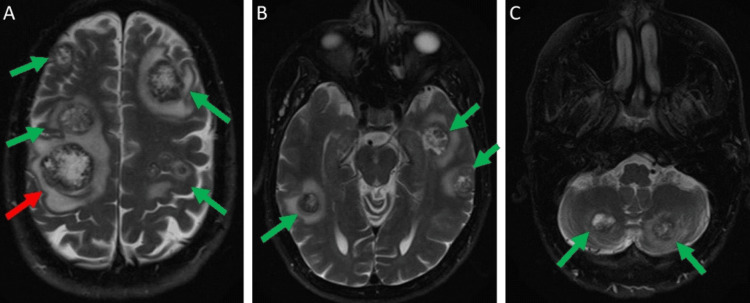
Initial MRI brain with IV contrast T2-weighted images. (A) Multiple brain lesions (green arrows) with significant surrounding edema involving bilateral frontal and parietal lobes, with the largest lesion measuring 2.9 cm in the right frontal lobe (red arrow). (B) Multiple brain lesions with surrounding edema (green arrows) involving bilateral temporal lobes. (C) Brain lesions (green arrows) with surrounding edema involving bilateral cerebellum. MRI: magnetic resonance imaging; IV: intravenous.
